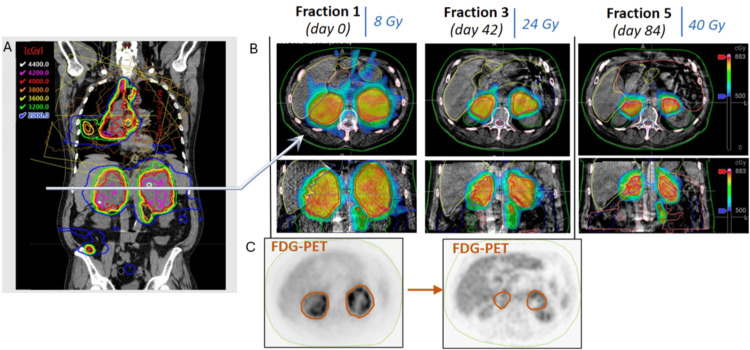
Base and adapted radiotherapy plans demonstrating interval response in bilateral adrenals. (A) Representative coronal image of the base radiotherapy plan for the multi-target plan. (B) Representative axial slices of bilateral adrenals demonstrating interval tumor shrinkage at fractions 3 and 5 from baseline. (C) Representative axial PET images demonstrating resolution of the FDG PET (fluorodeoxyglucose positron emission tomography) signal from baseline to prior to fraction 3.
